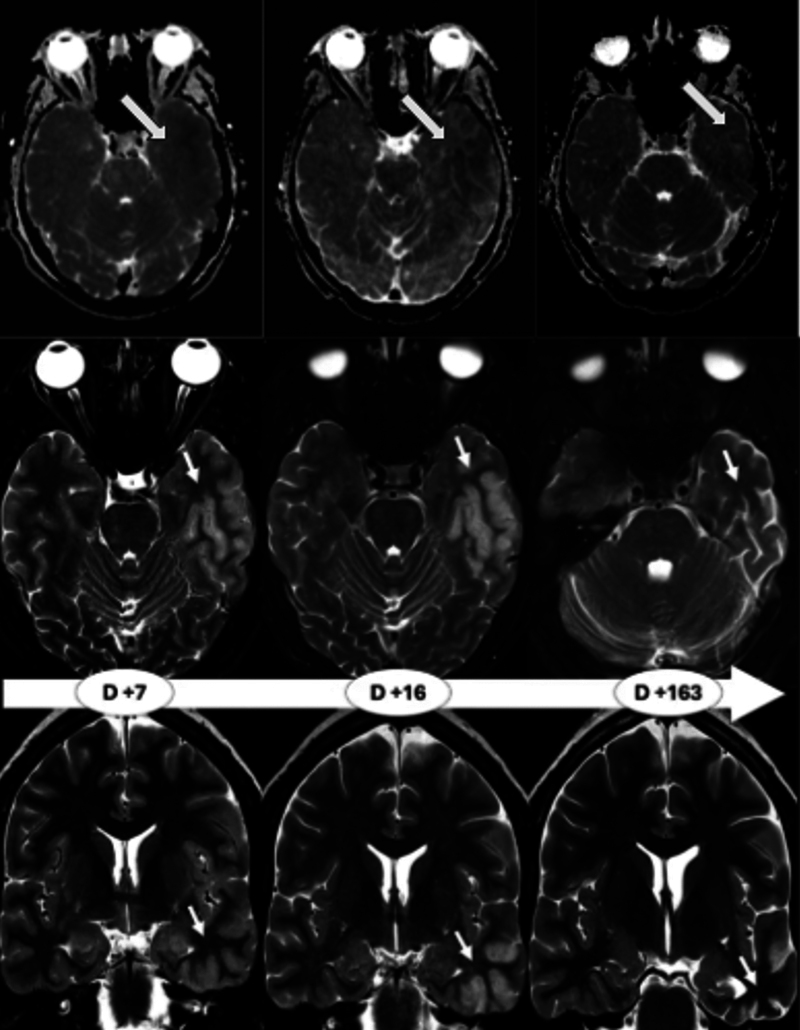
Follow-up magnetic resonance imaging (MRI) scans obtained at days 7, 16, and 163 demonstrates evolving cortical hyperintensities (arrows), which increase between days 7 and 16 and subsequently regress, with reexpansion of cortical sulci, parenchymal signal normalization, and residual temporal lobe atrophy. The middle and lower rows correspond to axial and coronal T2-weighted images, respectively. The upper row shows ADC maps demonstrating restricted diffusion, appearing as low signal intensity consistent with cytotoxic edema on days 7 and 16, with resolution of diffusion restriction by day 163, a pattern that may be observed following seizure activity.
